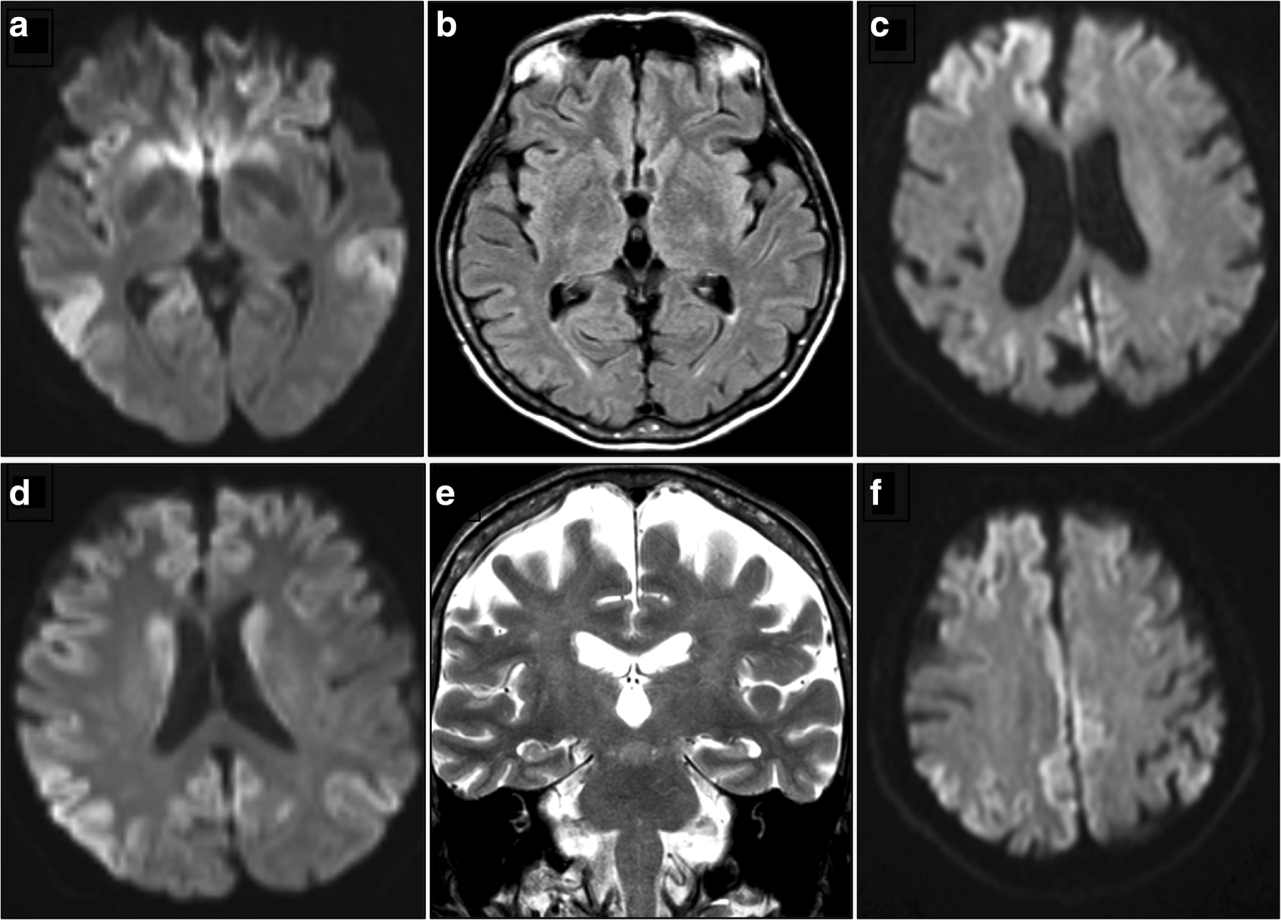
Imaging studies. a, d: DWI images show diffuse signal abnormalities involving bilaterally the posterior temporal cortex, caudate and putamen, parietal and frontal cortex, more marked in the right side (Case 1). b, e: Axial Flair and coronal T2-weighted images show diffuse cortical atrophy, involving frontal lobe with mild left prevalence (Case 2). c, f: DWI images show marked signal abnormalities in frontal and parietal right cortex and in right cingulus (Case 3)
